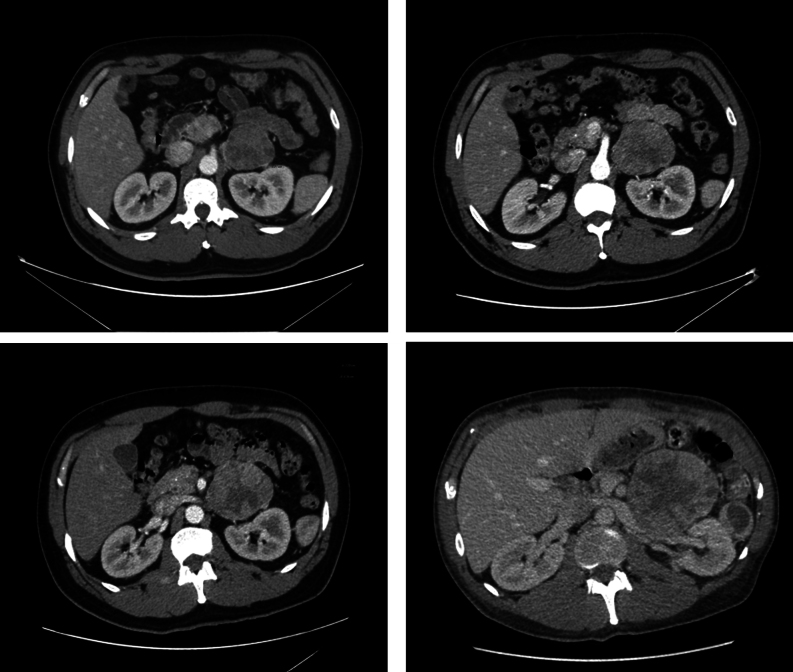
CT of the abdomen and pelvis, performed after the administration of intravenous material, demonstrated a progressively enlarging heterogeneous enhancing retroperitoneal mass thought to be arising from the tail of the pancreas, measuring up to 8.4 × 8.3 cm.
